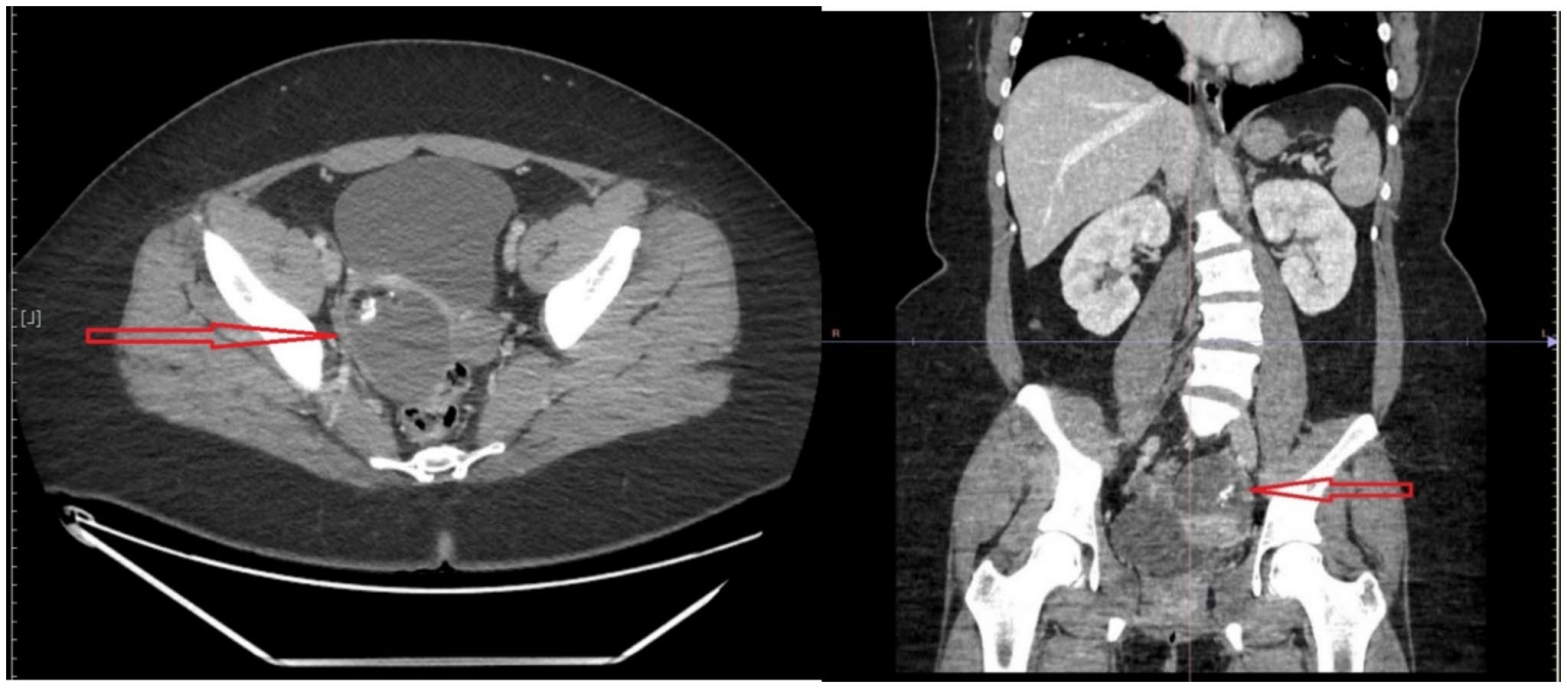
Computed tomography of the chest, abdomen and small pelvis with marked pathological mass of the left ovary.
